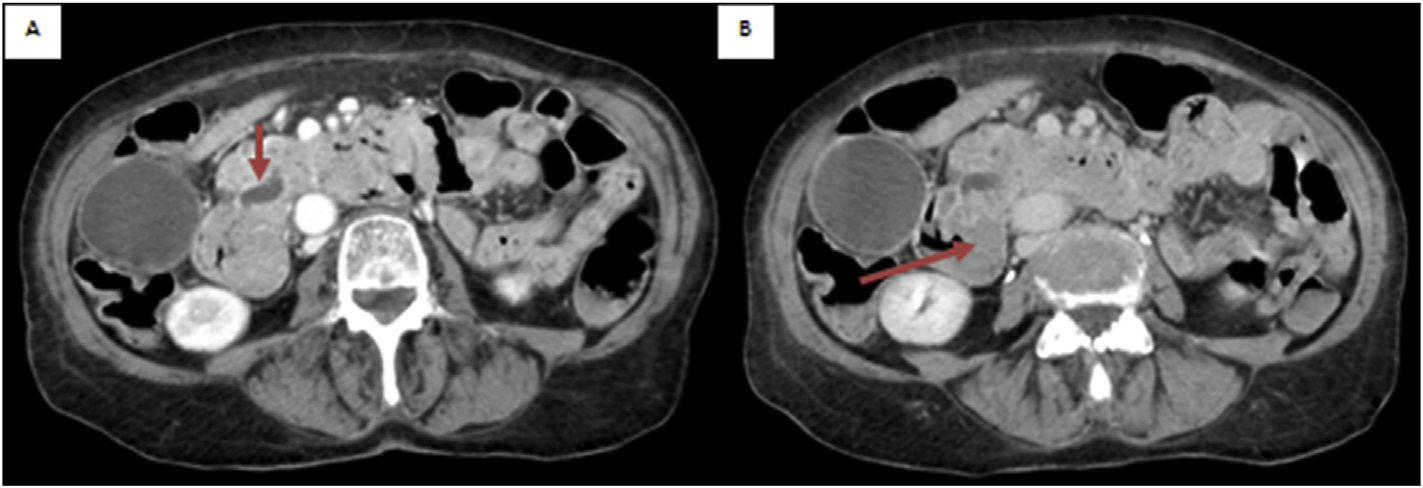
Duodenal adenocarcinoma shown on axial CT-scan during the portal phase (A and B). CT images show the marked thickening of the medial wall of the second part of the duodenum (B, arrow). The lesion had a central indentation and raised, rolled edges. In figure A (A, arrow) is evident the obstruction of the Vater papilla with dilatation of Wirsung duct.
